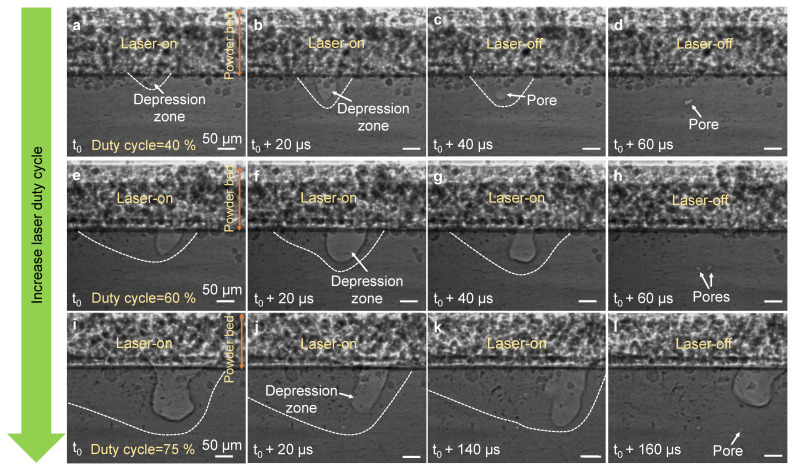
Pore formation under varying laser duty cycles at a constant laser frequency: (a–l) Dynamic X-ray images showing pore formation during the PW-LPBF process of Al6061 at the laser duty cycle of 40% (a–d), 60% (e–h), and 75% (i–l) at a laser frequency of 7 kHz, a laser power of 470 W and a scan speed of 0.4 m/s. In (d,h,l) the melt pool boundary is not indicated to avoid blocking the view of the pores. Note that images do not display the complete duration of one pulse. The compiled movies showing the complete duration of two consecutive pulses are available in the Supplementary Material (Movies S4–S6).
